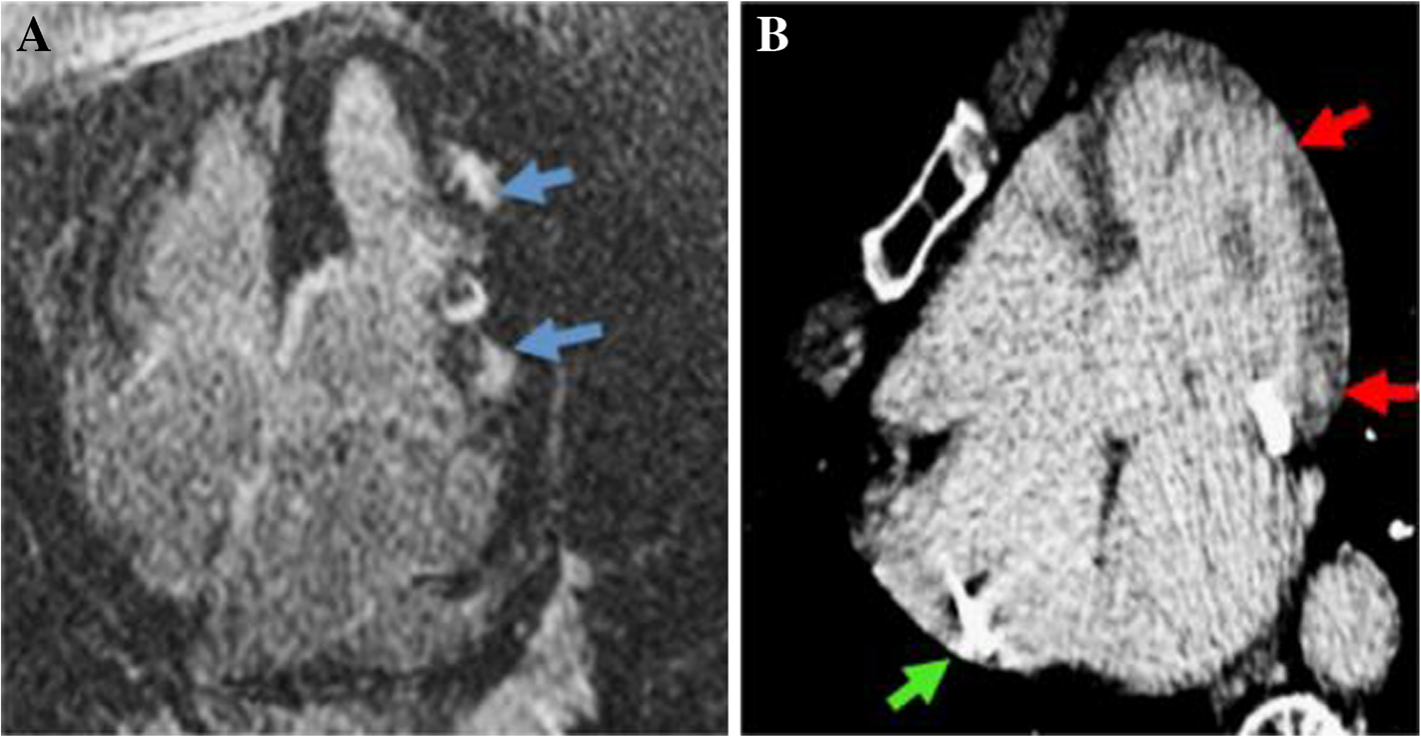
Panel A. 72 year old female with known sarcoidosis was found to have patchy areas of delayed hyper enhancement (blue arrows) in the lateral wall of the left ventricle at cardiac MRI. Panel B. Three months after pacemaker placement which precluded repeat MRI (green arrow), the patient developed symptoms warranting analysis for cardiac sarcoid progression. MDE by cardiac CT shows corresponding patches of delayed hyperenhancement (red arrows) which confirmed stable involvement since the recent cardiac MRI.
